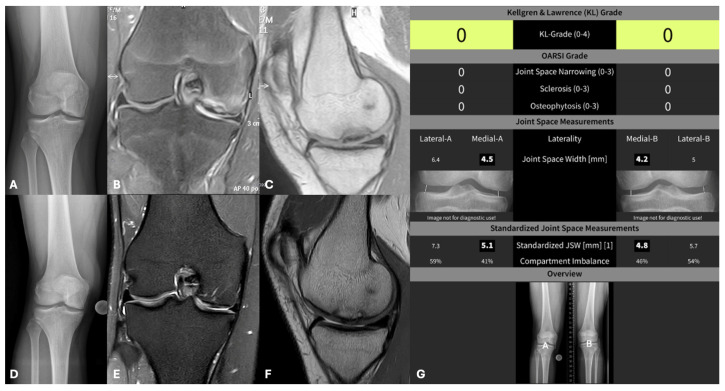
(A–G): Patient (16 years old) with OCD at the medial femoral condyle on the right knee before surgery, native X-ray (A); preoperative MRI scans (B,C); 6-year follow-up after OCD pinning with X-ray (D) and postoperative MRT scans (E,F); medial and lateral automatized joint space width evaluation of the right and contralateral left knee at defined measurement points (G).
